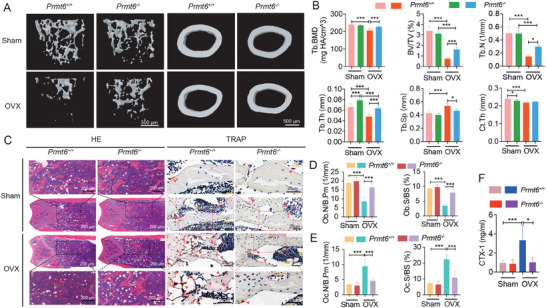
Prmt6* deficiency attenuates OVX‐induced bone loss and osteoclastogenesis in vivo. A) Micro‐CT images from *Prmt6^−/−^
